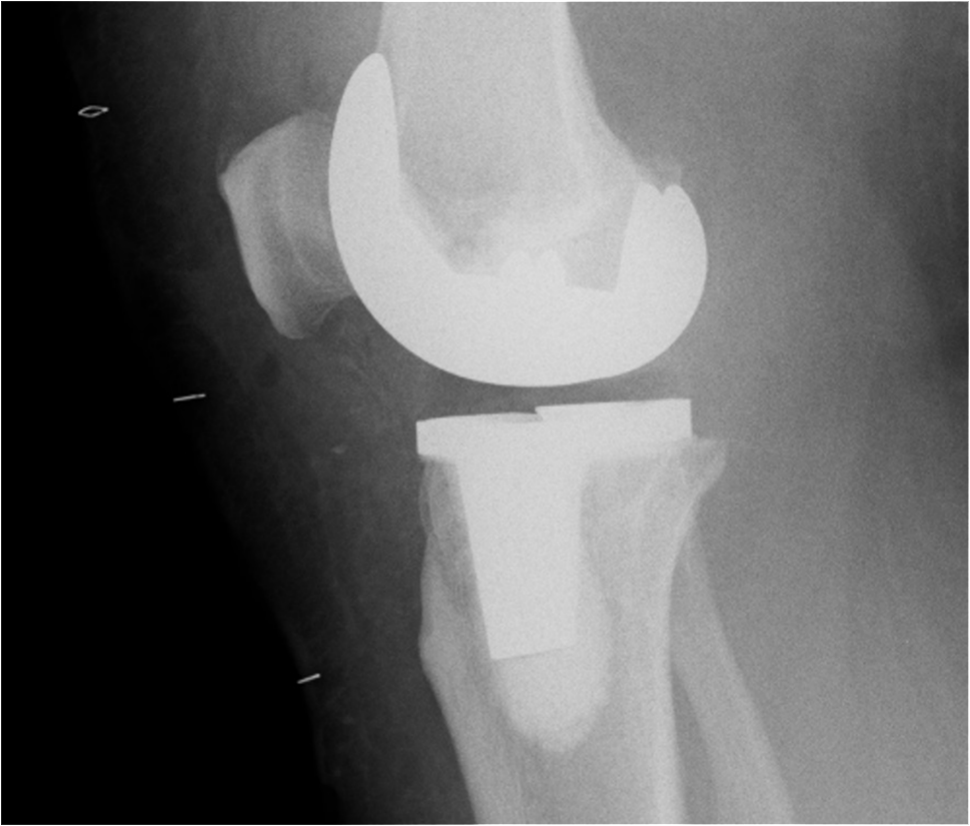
An immediate post-operative lateral radiograph of a non-augmentable TKA. As visible here, we noted the consistent presence of a radiolucent line at the implant/cement interface at the tip of the keel
